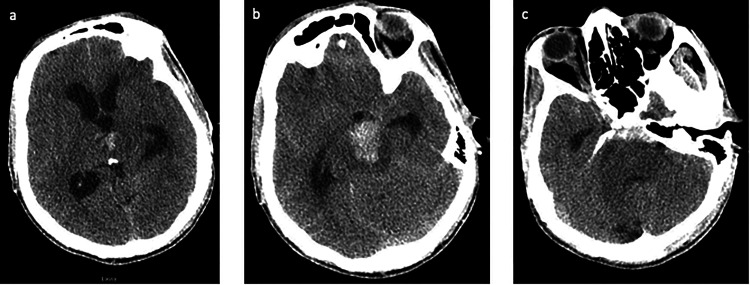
A 51-year-old male (patient 1) presenting with headache and neurological decline. Axial view of the noncontrast head CT showing (a) IVH with obstructive hydrocephalus, (b) pontine ICH, and (c) SAH in the prepontine cistern. CT: computed tomography; ICH: intracerebral hemorrhage; IVH: intraventricular hemorrhage; SAH: subarachnoid hemorrhage.
